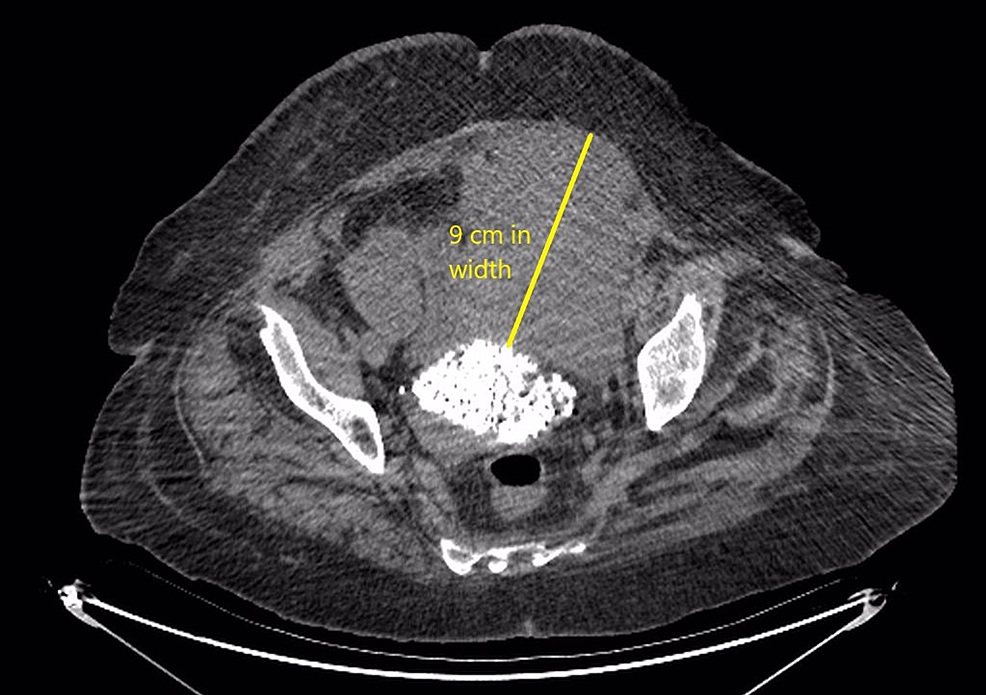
Abdominal and pelvic CT showed a left inferior rectus sheath hematoma reaching approximately 9 cm in width.
